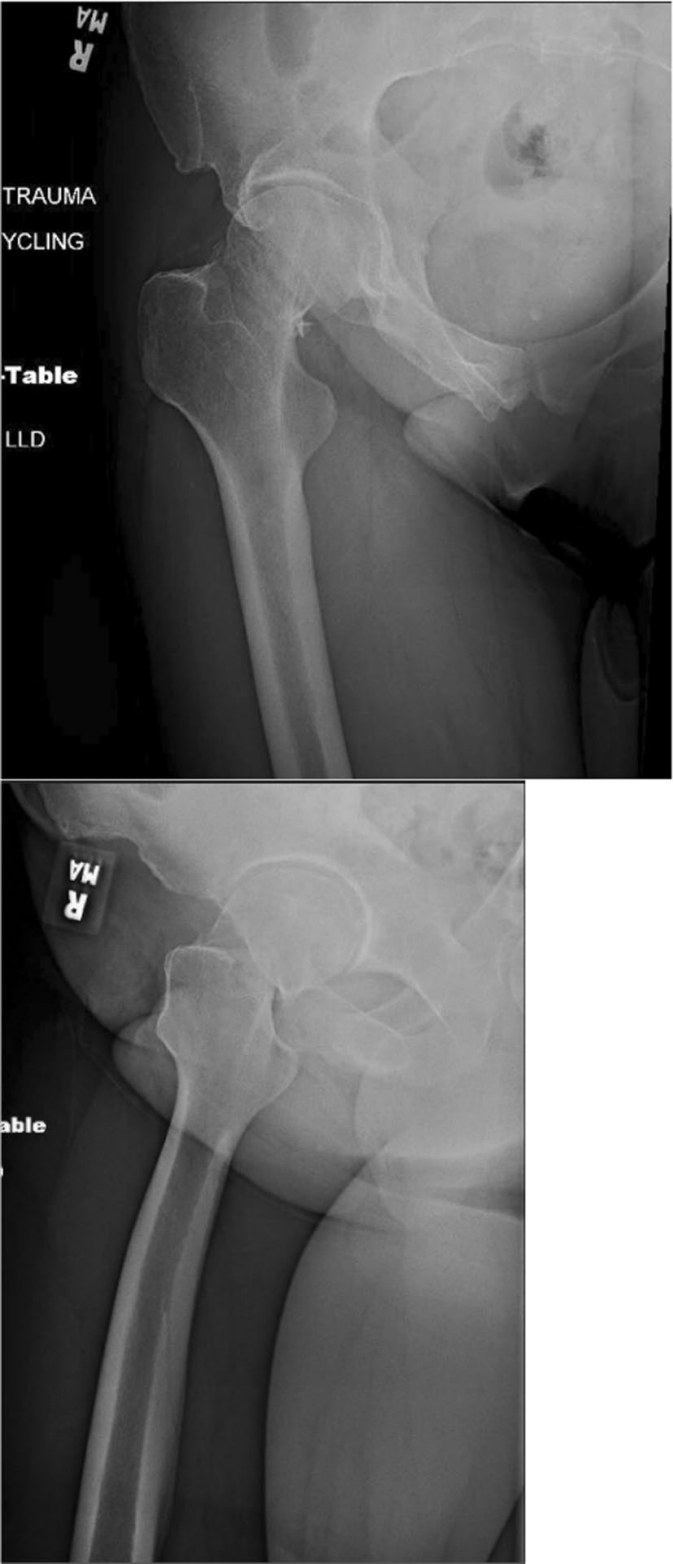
Pre-operative X-ray images of the clinical vignette provided by OrthoBullets
